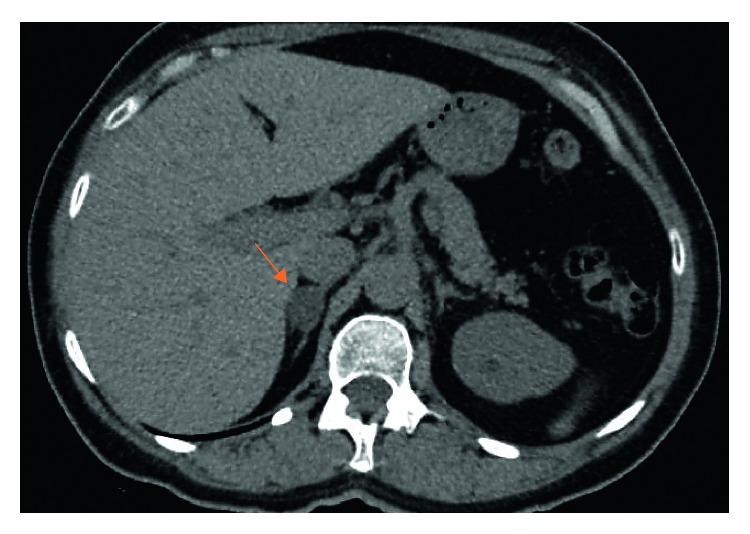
Abdominal computed tomography (CT) scan showing a 2.5 cm adrenal nodule suggestive of adenoma on the right (arrow).
